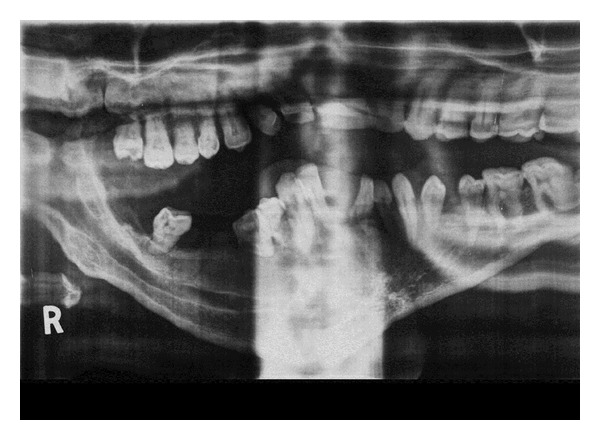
Panoramic radiograph showing widening of the right inferior alveolar canal.
